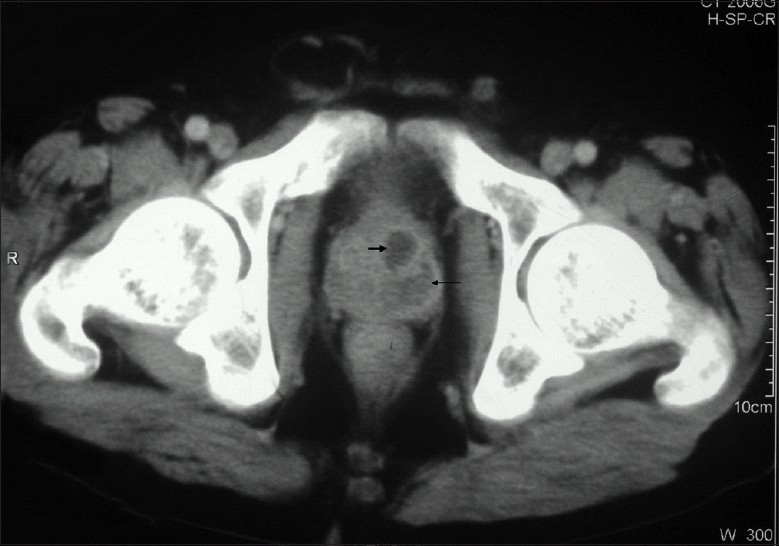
CT scan showing hypodense lesions in the prostate
